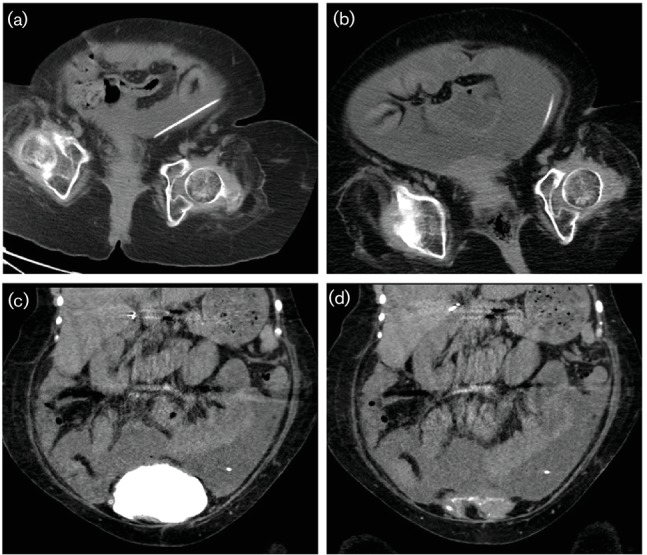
(a, b) Axial cross-section from CT of the abdomen and pelvis demonstrating: small volume pelvic fluid collection 1 year prior to the illness (a) and large pelvic fluid collection at the time of admission (b). (c, d) Coronal cross-section from contrasted CT cystogram demonstrating no detectable extravesicular dye in the abdomen and pelvis on admission: pre-void (c) and post-void (d).
